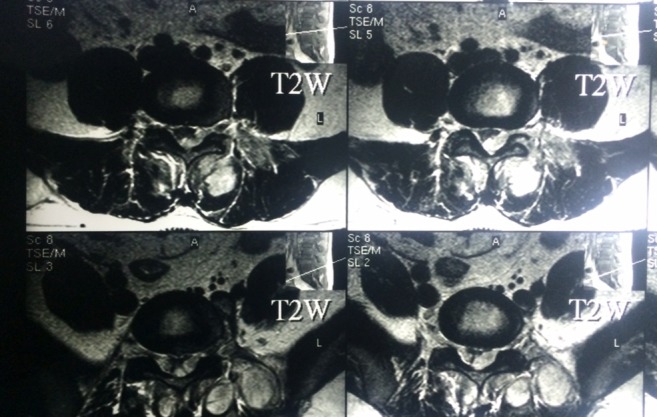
Preoperative MRI, axial section T2W, show multiple pockets of pus in the paraspinal muscles.
